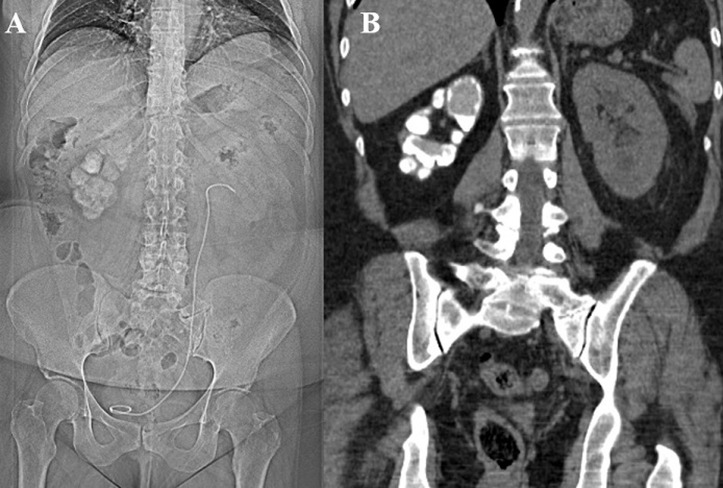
(A) CT scout view (topogram, instead of X-ray kidney, ureter and bladder) revealing diffuse alveolar calcification with kidney shape in right renal region and a well-positioned pigtail catheter; (B) computed tomography image showing the densely calcified right kidney
